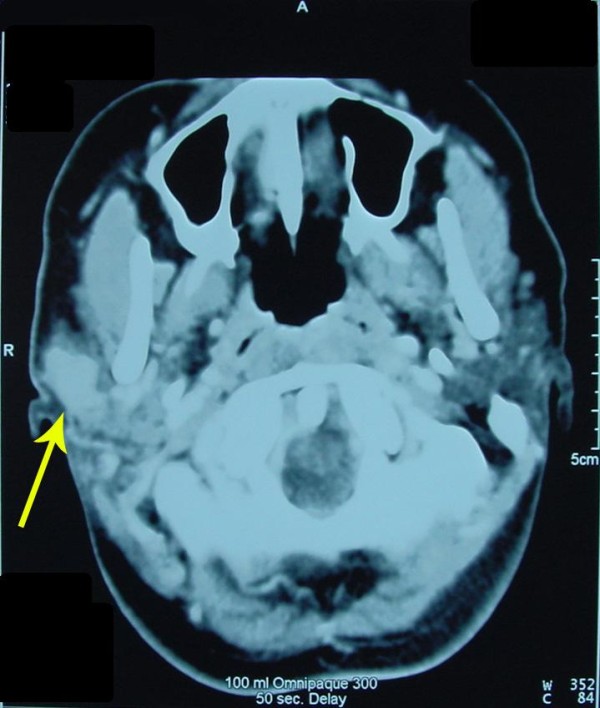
An axial CT-scan image confirming the presence of a solid mass lesion (acinic cell carcinoma) within the right parotid gland.
